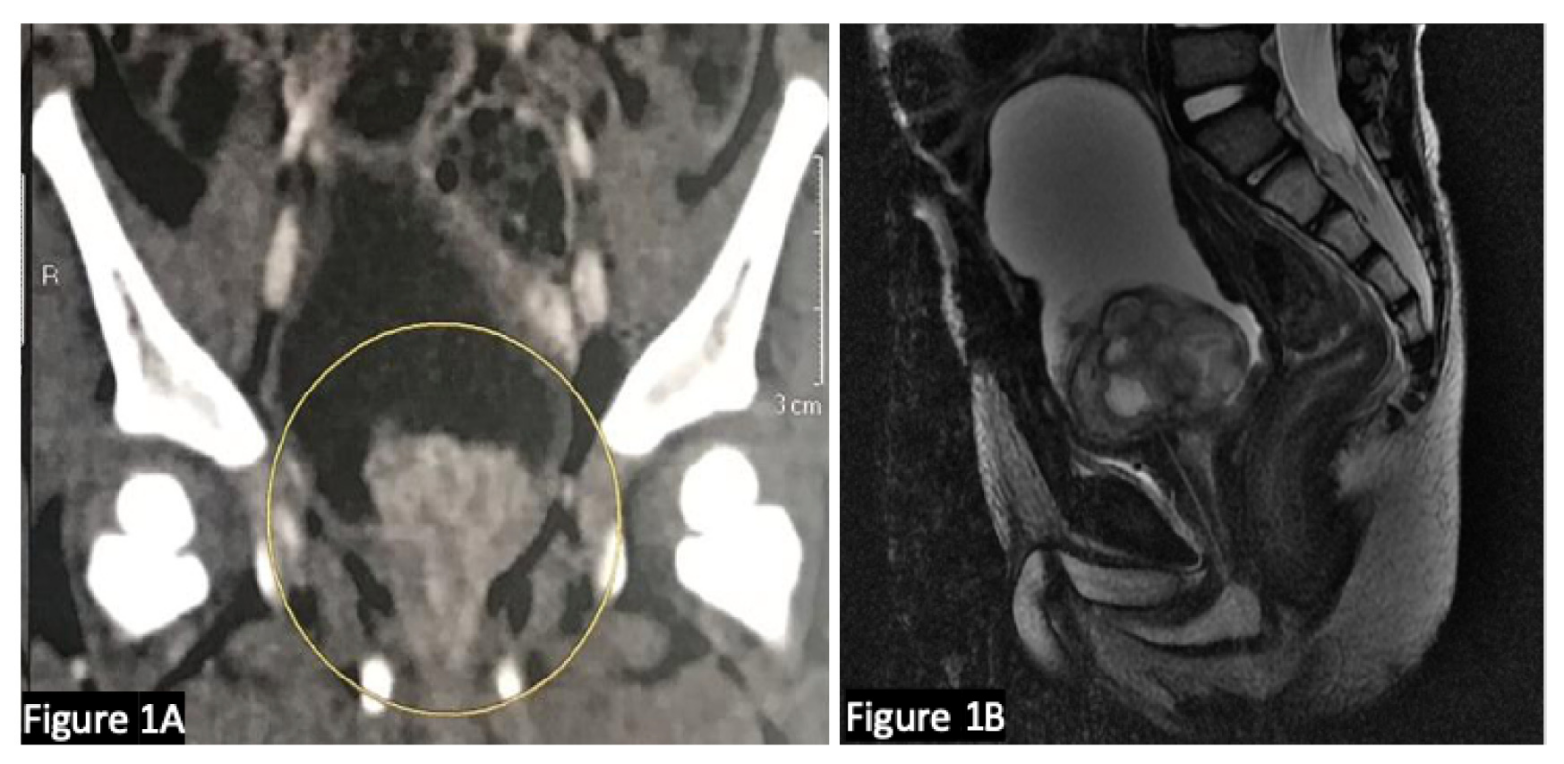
Computed tomography and magnetic resonance images of tumor. (
